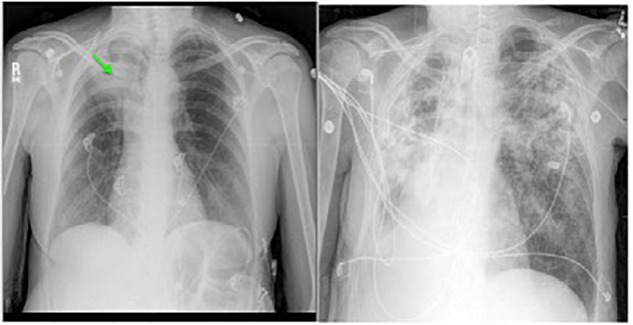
Left: chest radiograph showing right upper lobe consolidation with central cavitary changes (green arrow) in a woman with COPD and pulmonary NTM disease. Right: chest radiograph of same subject demonstrating marked progression of pulmonary NTM disease, including likely spillage of the previous right upper lobe cavitary lesion.
